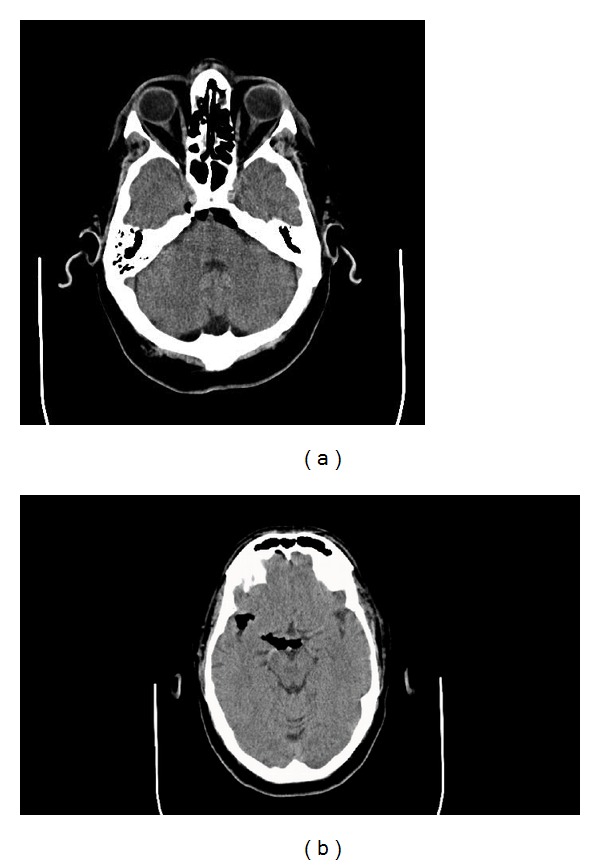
Noncontrast CT scan of the brain displaying significant pneumocephalus exerting slight mass effect on the pons.
